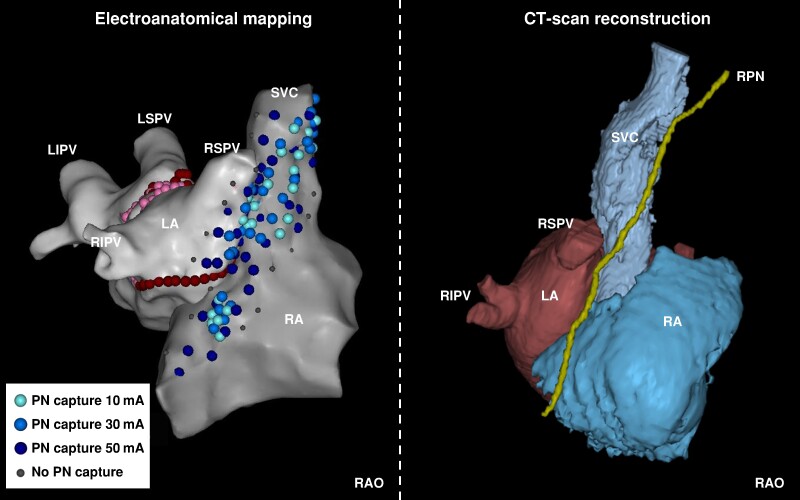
Left panel: electroanatomical mapping of the right and left atrium with RPN pace-mapping sites, colour-labelled with the RPN capture threshold. Right panel: corresponding 3D reconstruction of the CT scan, with the course of the RPN segmented in yellow. LA, left atrium; LIPV, left inferior pulmonary vein; LSPV, left superior pulmonary vein; RA, right atrium; RIPV, right inferior pulmonary vein; RPN, right phrenic nerve; RSPV, right superior pulmonary vein; SVC, superior vena cava.
